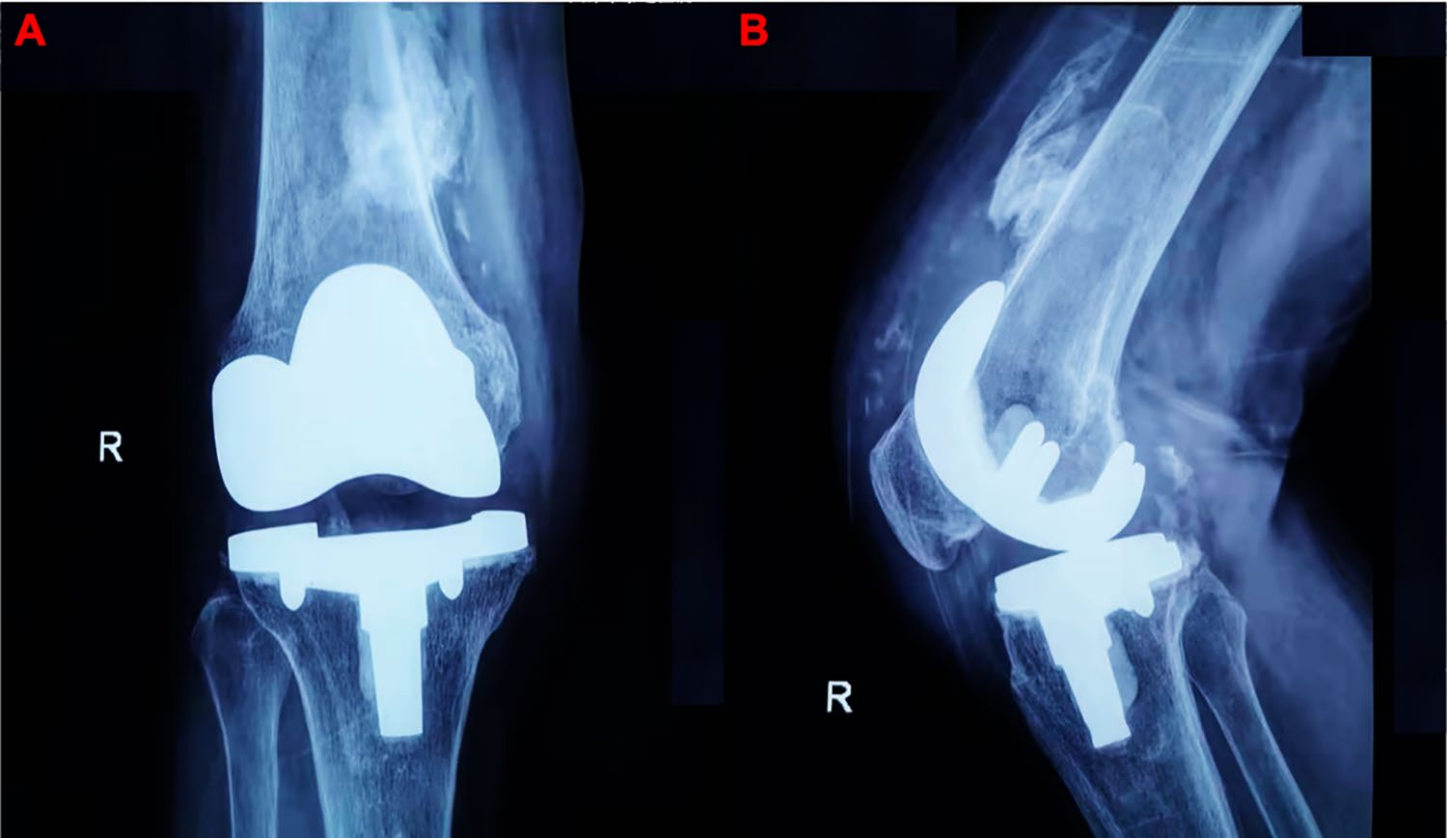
Postoperative TKR AP view (A) and lateral view (B) radiograph of patient, showing type III HO (more than 5 cm of new bone formation).
